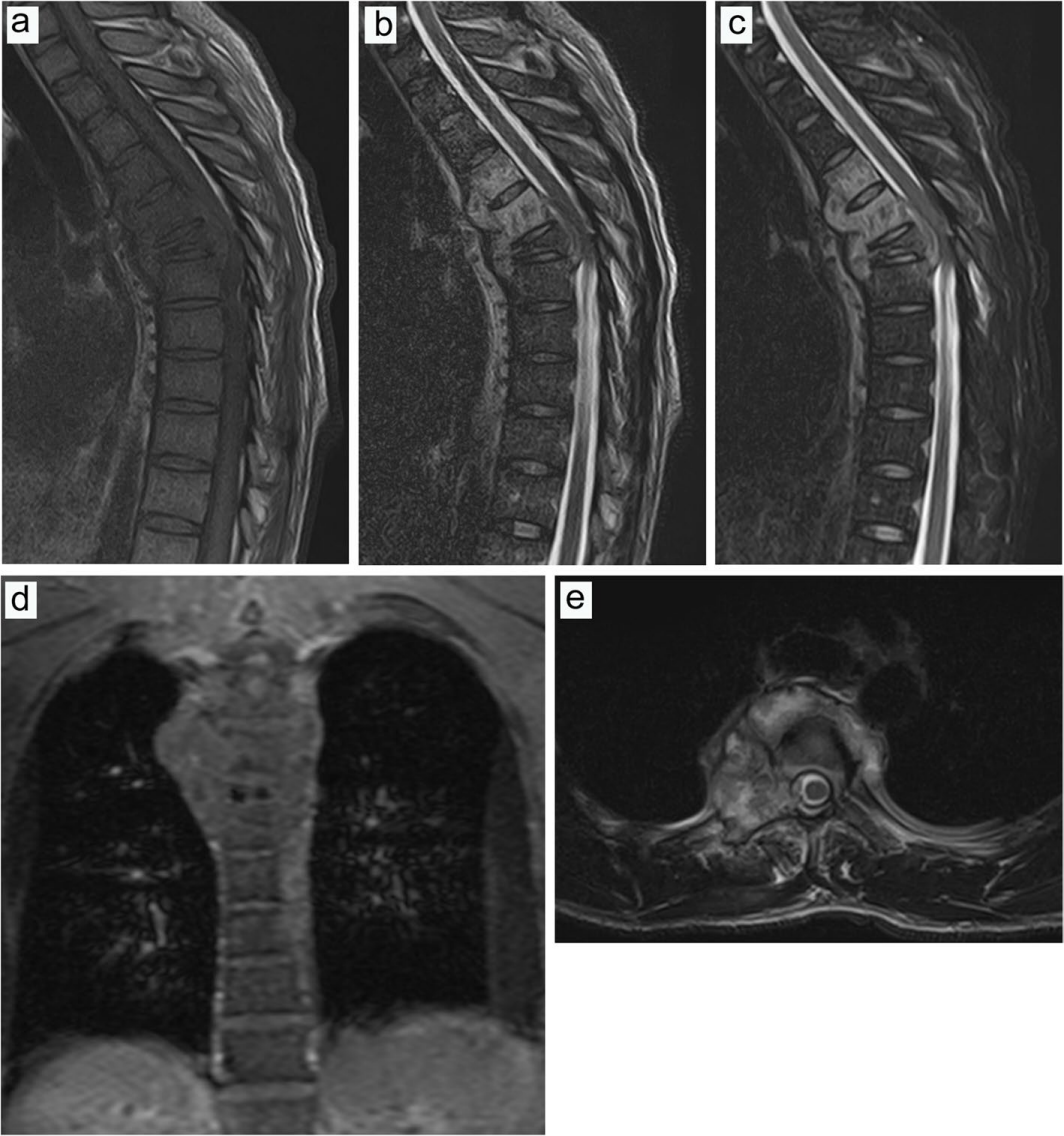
Preoperative MRI examination in one patient without intraoperative blood transfusion. a, MRI examination in the sagittal T1 sequence. b, MRI examination in the sagittal T2 sequence. c, MRI examination of T2 lipid compression sequence in the sagittal position. d, MRI examination in the coronal T2 sequence. e, MRI examination of the T2 sequence in cross-section
